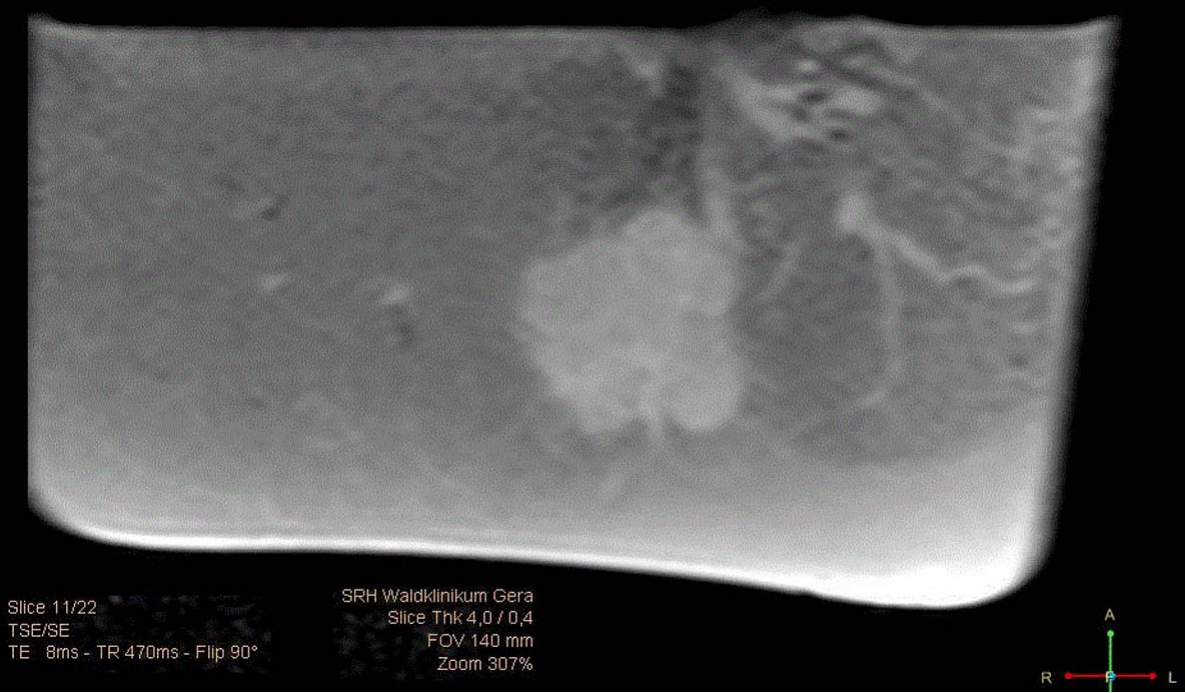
Flooded lung lobe containing NSCLC in T1w MR image
